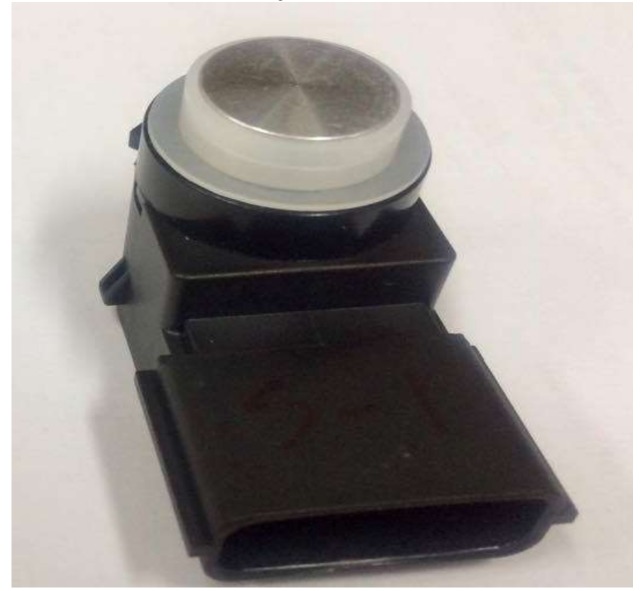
An actual ultrasonic sensor for in-air applications.
